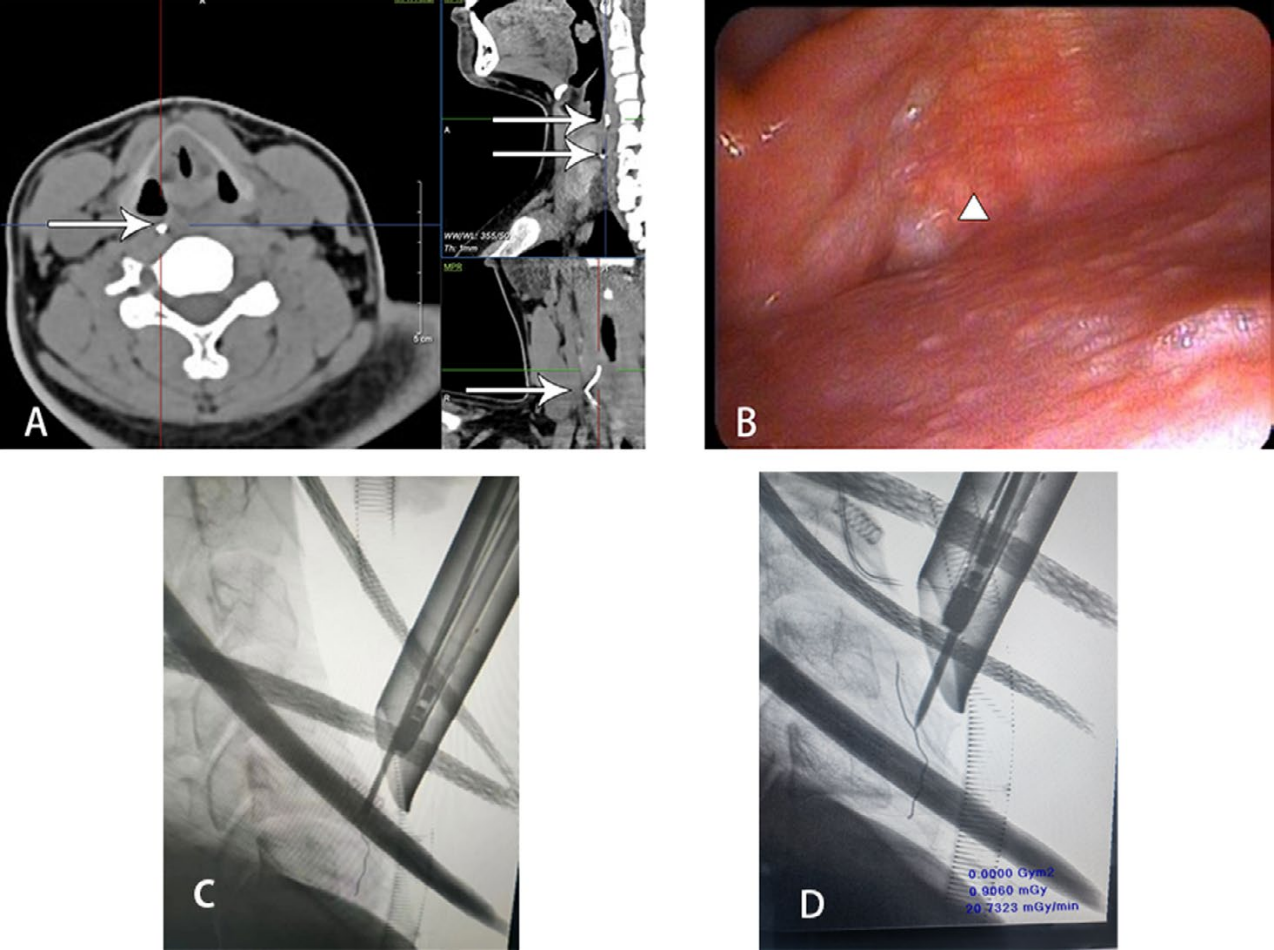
Imaging examination of the patient. (A) Neck CT confirmed the presence of an obliquely oriented density (arrow) in the retropharyngeal space at the C4–5 level. (B) The electronic laryngoscope image showed a small area of suspected protuberance was noted on the right piriform fossa (triangle). (C) The first lateral neck intraoperative X‐ray. (D) The second lateral neck intraoperative X‐ray.
